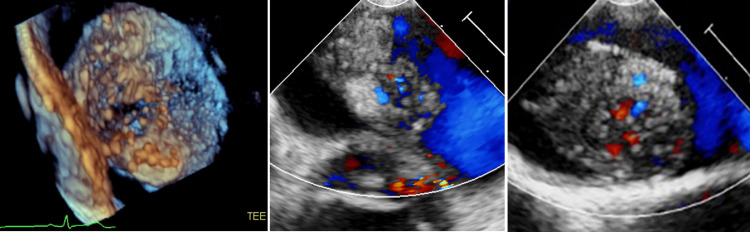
Images from TEE demonstrating irregular borders and varying echogenicity in 3D as well as color flow highlighting vascularity within the tumor TEE: transesophageal echocardiogram
